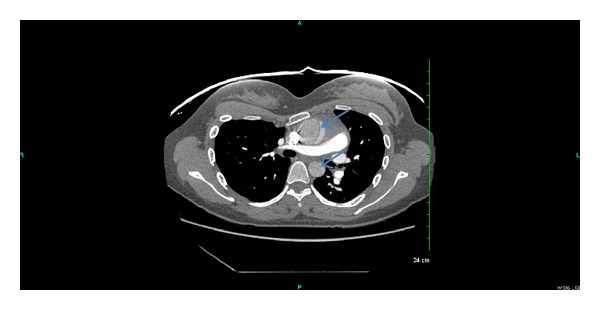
CT chest with IV contrast showing intimal flap (arrows) in both ascending and descending aortae.
